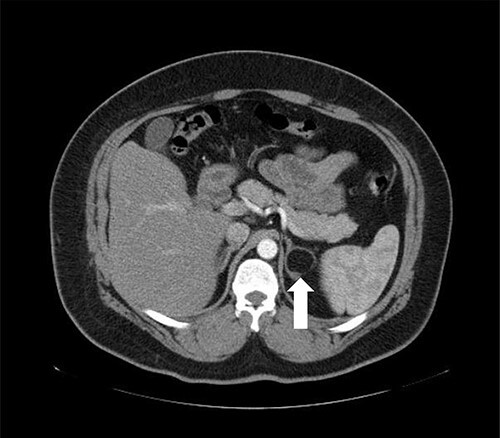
Axial CT of the abdomen showing the left adrenal mass in Patient 3 (white arrow).
